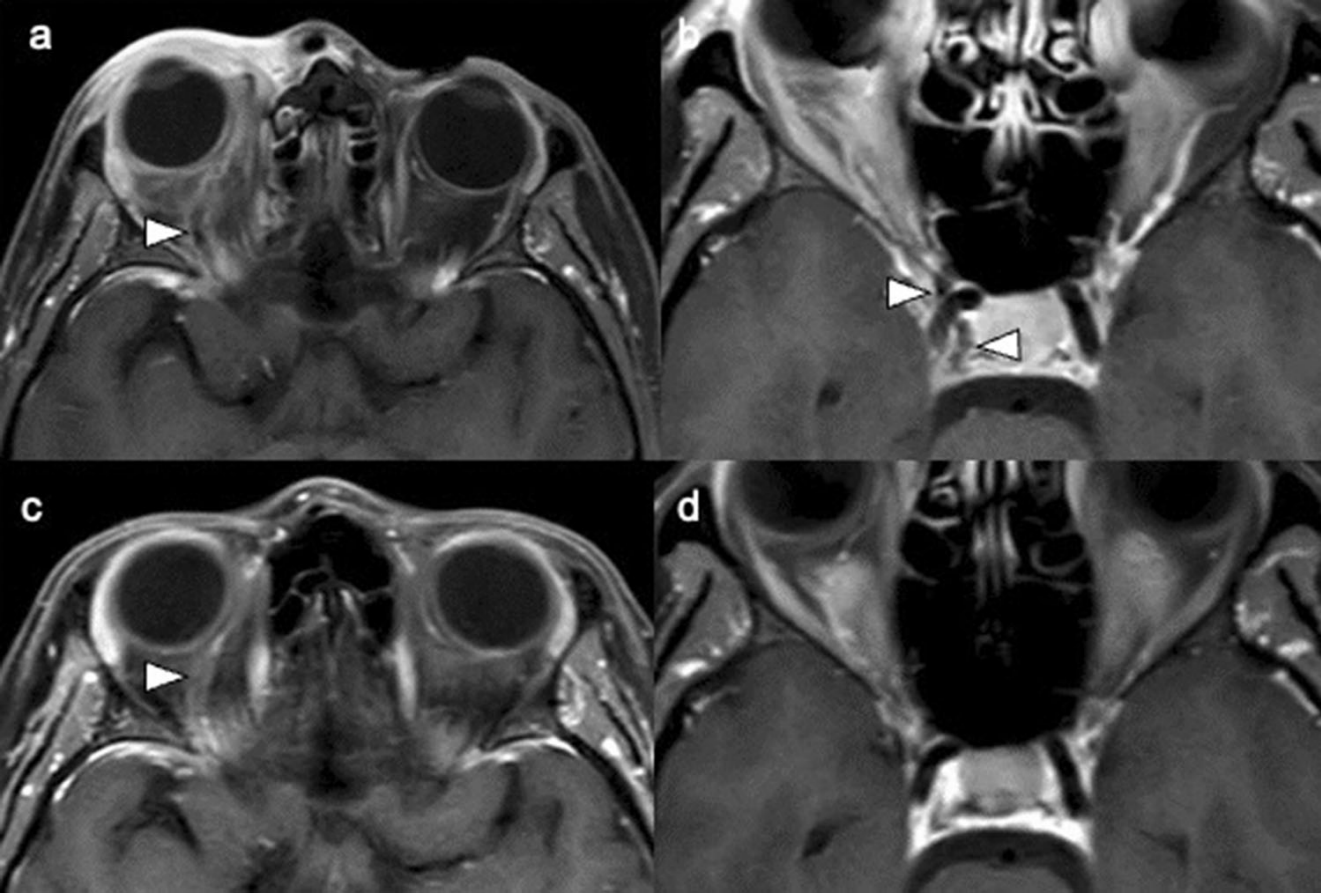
Contrast-enhanced fat-saturated T1-weighted magnetic resonance imaging of the orbit showed a, b filling defects in the dilated right superior ophthalmic vein and cavernous sinus (arrowhead). c, d The filling defects had partially resolved on follow-up imaging
